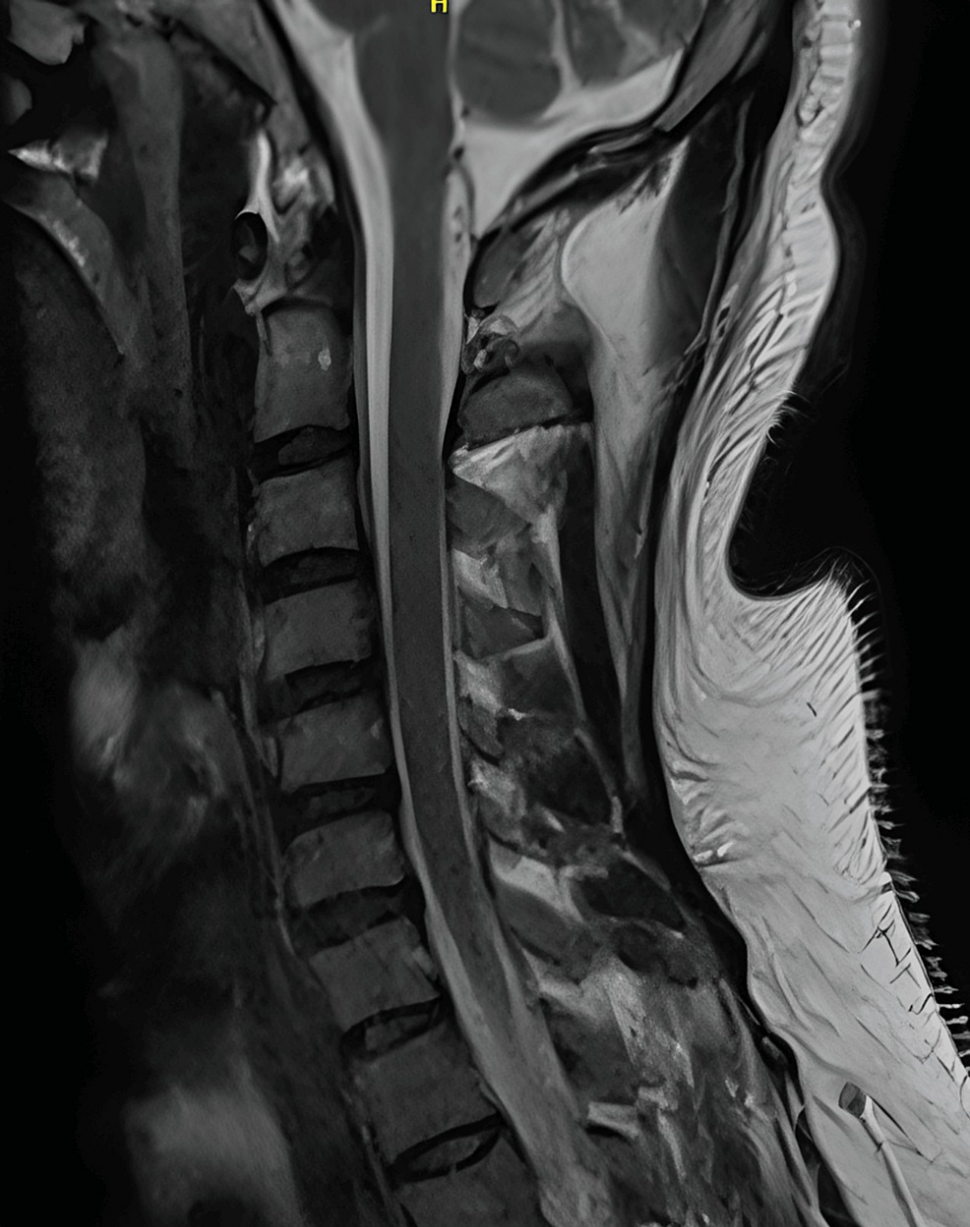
MRI Cervical Spine (T2-Weighted Sagittal Section) MRI Cervical Spine: The cervical vertebrae appear normal in height, contour, and signal intensity.  Cranio-cervical junction appears normal.  No focal bony lesion could be seen. The intervertebral discs are confined to the outlines of the corresponding endplates and vertebral bodies and maintain normal height and signal intensity. The cervical spinal cord appears normal in diameter and signal intensity.
